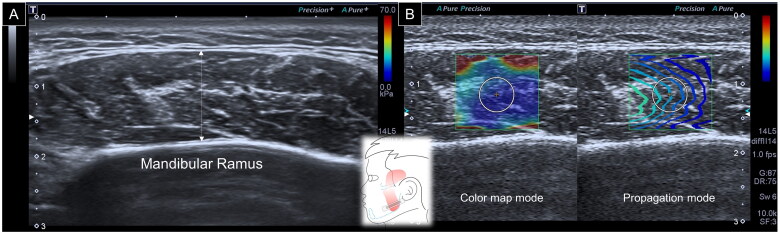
Ultrasound imaging for thickness (A) and stiffness (B) measurements of the masseter muscle. White dashed line, maximal muscle thickness.
